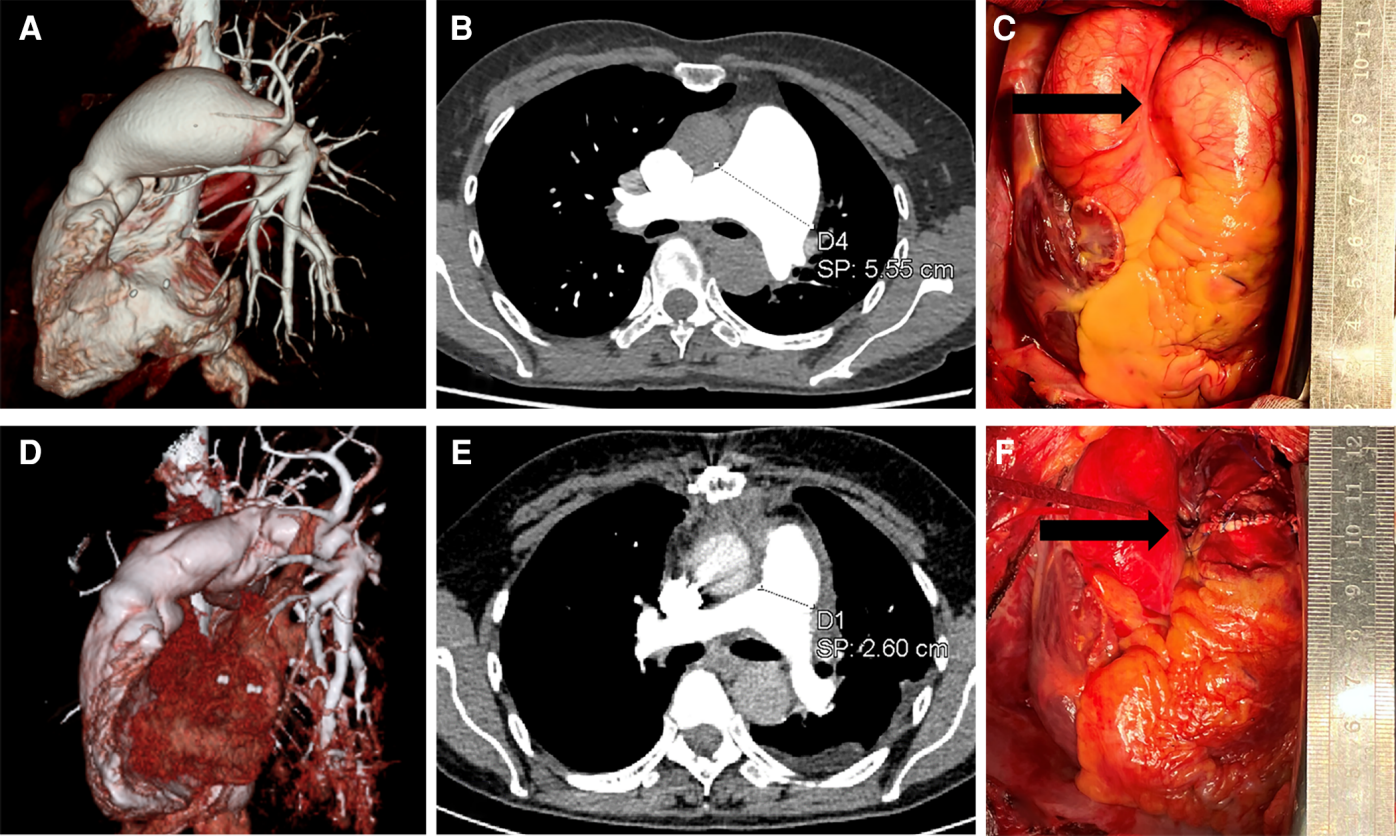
Preoperative and postoperative data. (A,B) are preoperative pulmonary artery CTA images showing an aneurysmal dilation of the pulmonary artery, with a maximum width of 5.55 cm. (D,E) are postoperative pulmonary artery CTA images demonstrating restoration of normal diameter in the main pulmonary artery, approximately 2.6 cm. Images (C,F) are intraoperative images, with (C) showing the pulmonary artery aneurysm under direct visualization, and (F) displaying the pulmonary artery after vessel replacement.
